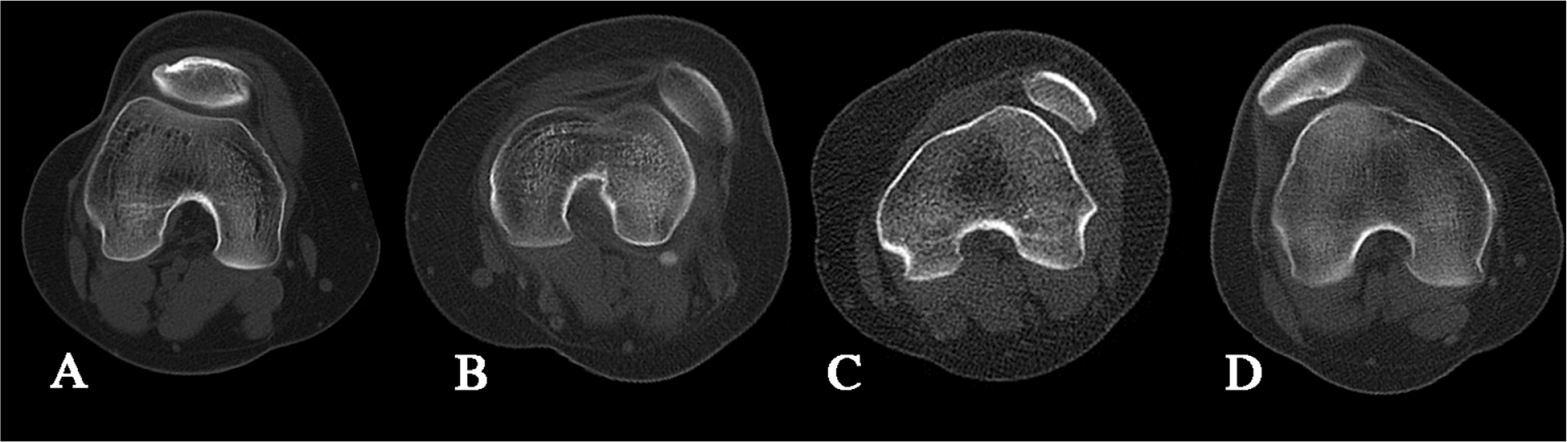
The Dejour classification. A CT showed the Dejour classification of trochlear dysplasia, type A. B CT showed the Dejour classification of trochlear dysplasia, type B. C CT showed the Dejour classification of trochlear dysplasia, type C. D CT showed the Dejour classification of trochlear dysplasia, type D
